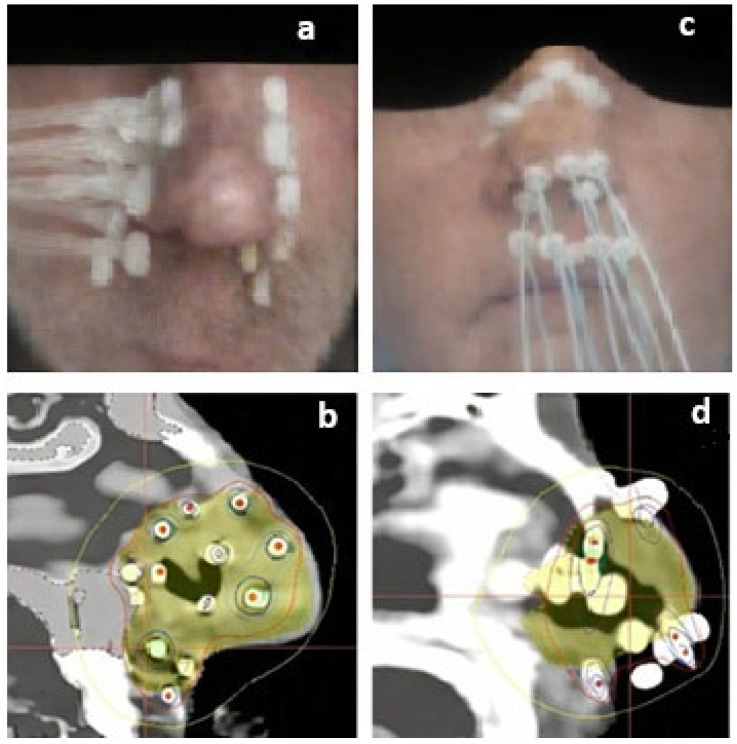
Interstitial HDR-IRT catheters implanted with a representative sagittal CT image showing the dose distribution; (a,b): transversely, (c,d): longitudinally. The clinical target volume (CTV) is displayed in yellow, and the 100% prescription isodose line is represented by the red contour.
